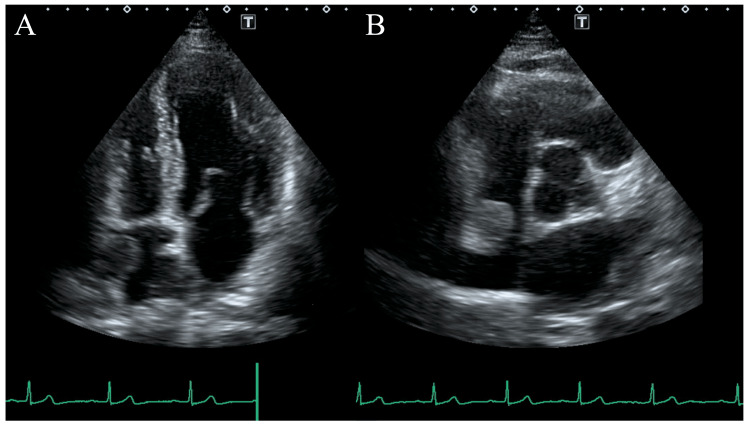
Transthoracic echocardiography. A) Four-chamber view. A round, rough surface mass was present in the right atrium; the area surrounding the mass was highly echoic, while the internal area was slightly low-echoic. B) Short axis view, aortic valve level.
